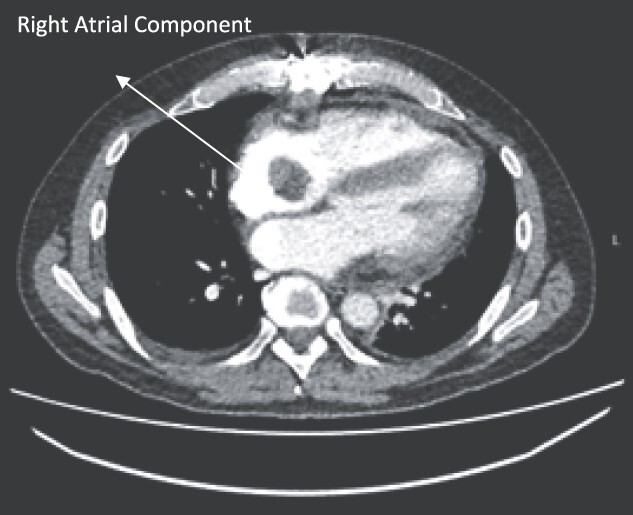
Post-operative CT scan during chemotherapy showing reduction of size RA bulk.
